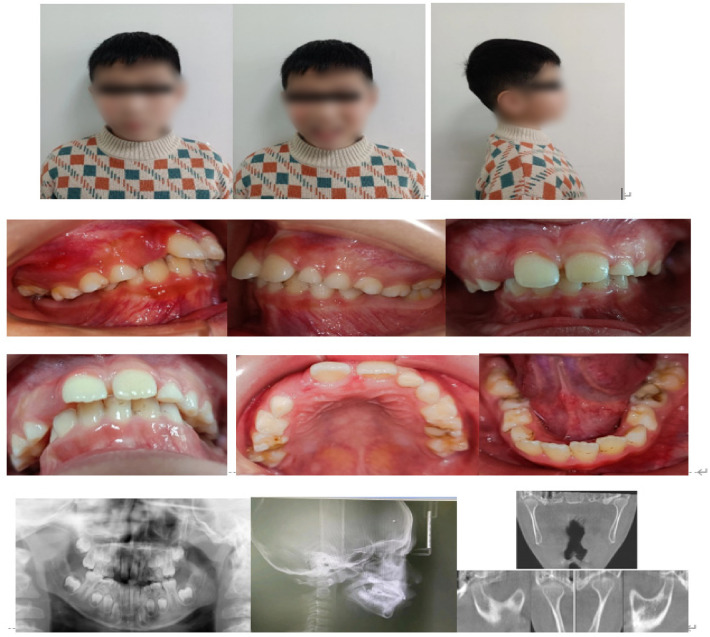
Extraoral frontal view, intraoral occlusal view and radiographic images before treatment.
